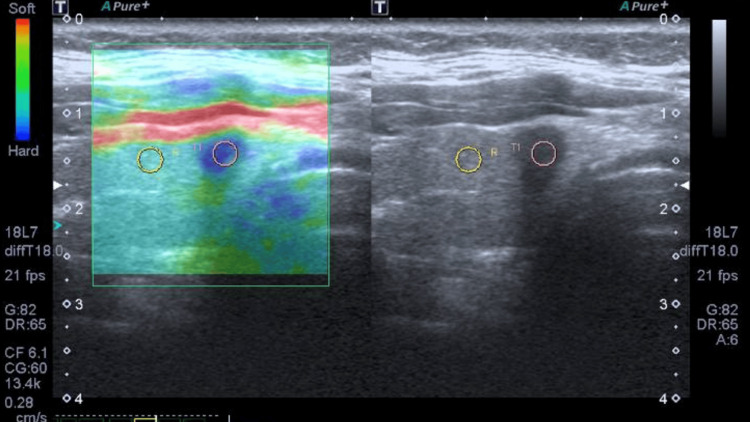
Ultrasound elastography. A 48-year-old male patient with a right thyroid nodule classified as ACR TI-RADS 5. Quantitative elastography score was 5, and qualitative elastography A score was 4. The pathology report was suspicious for papillary carcinoma, Bethesda category V. ACR TI-RADS: American College of Radiology Thyroid Imaging Reporting and Data Systems.
